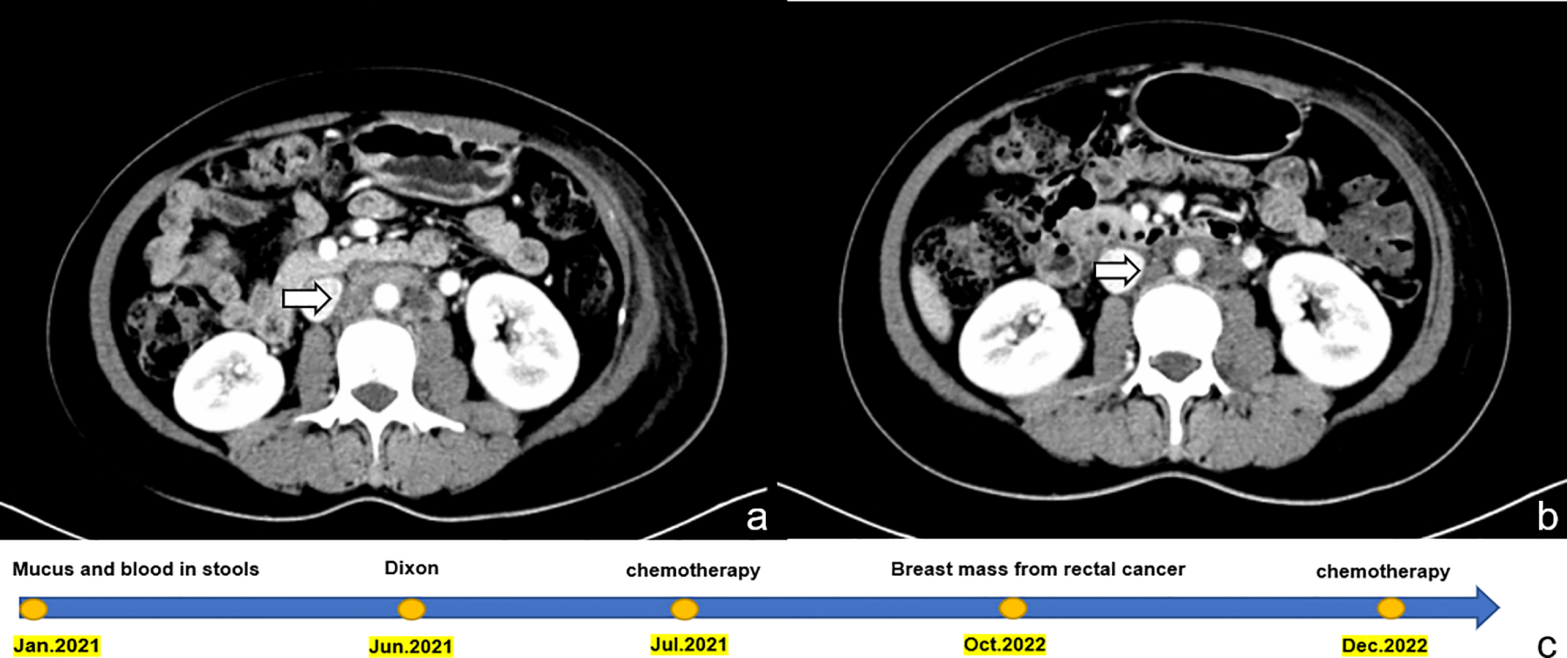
Shows a comparison of abdominal CT images before and after chemotherapy in the patient, and a timeline of the patient’s treatment process. (A) displays the image prior to chemotherapy, with enlarged lymph nodes indicated by the arrow. (B) displays the image after three courses of chemotherapy, with the same lymph nodes indicated by the arrow now visibly reduced in size. This visual representation highlights the effectiveness of chemotherapy in reducing the size of the lymph nodes. (C) timeline of the patient’s treatment process.
